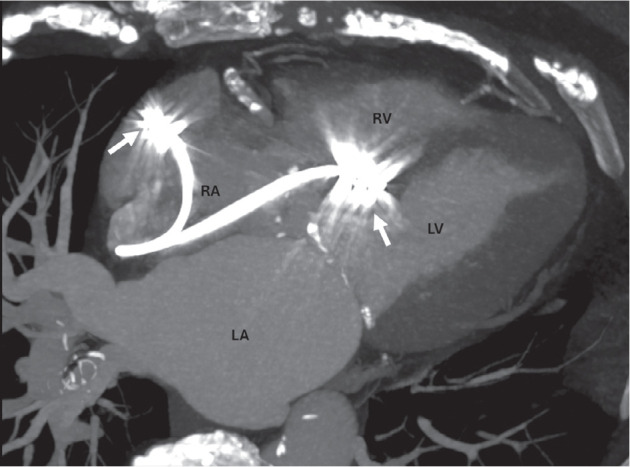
Contrast-enhanced cardiac computed tomography scan with maximal-intensity projection reformation in four-chamber view showing the pacemaker leads located in the right atrium and right ventricular septum (arrows). *Abbreviations:* LA, left atrium; LV, left ventricle; RA, right atrium; RV, right ventricle.
